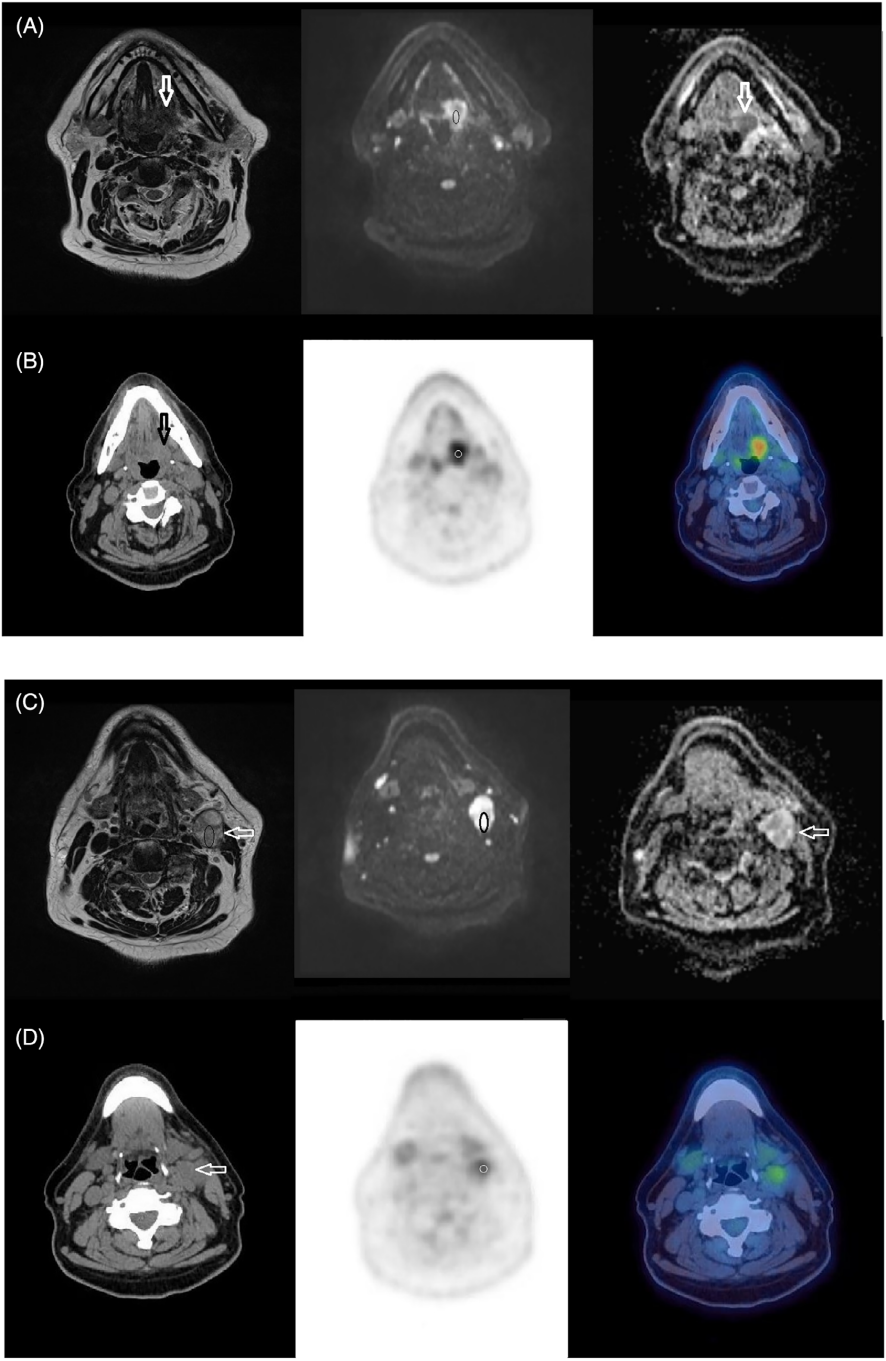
Pretreatment MRI and FDG PET/CT study in a 64‐year‐old male patient with T2N2b left oropharynx tumor. T2W, *b* = 800 DWI and ADC map (*b* = 100‐800) MRI images (A and C) and CT, PET, PET/CT fused images (B and D) indicating the left glosso‐tonsillar sulcus tumor (arrows in A and B) and left level 2 lymph node (arrows in C and D). The ROIs on the MRI study include areas of increased DWI signal corresponding to intermediate T2w signal in the cores of the primary and nodal tumor as indicated (ovals in A and C). The 6 mm VOI on the PET‐CT study is seen within the central portion of the primary and nodal tumor (circles in B and D)
